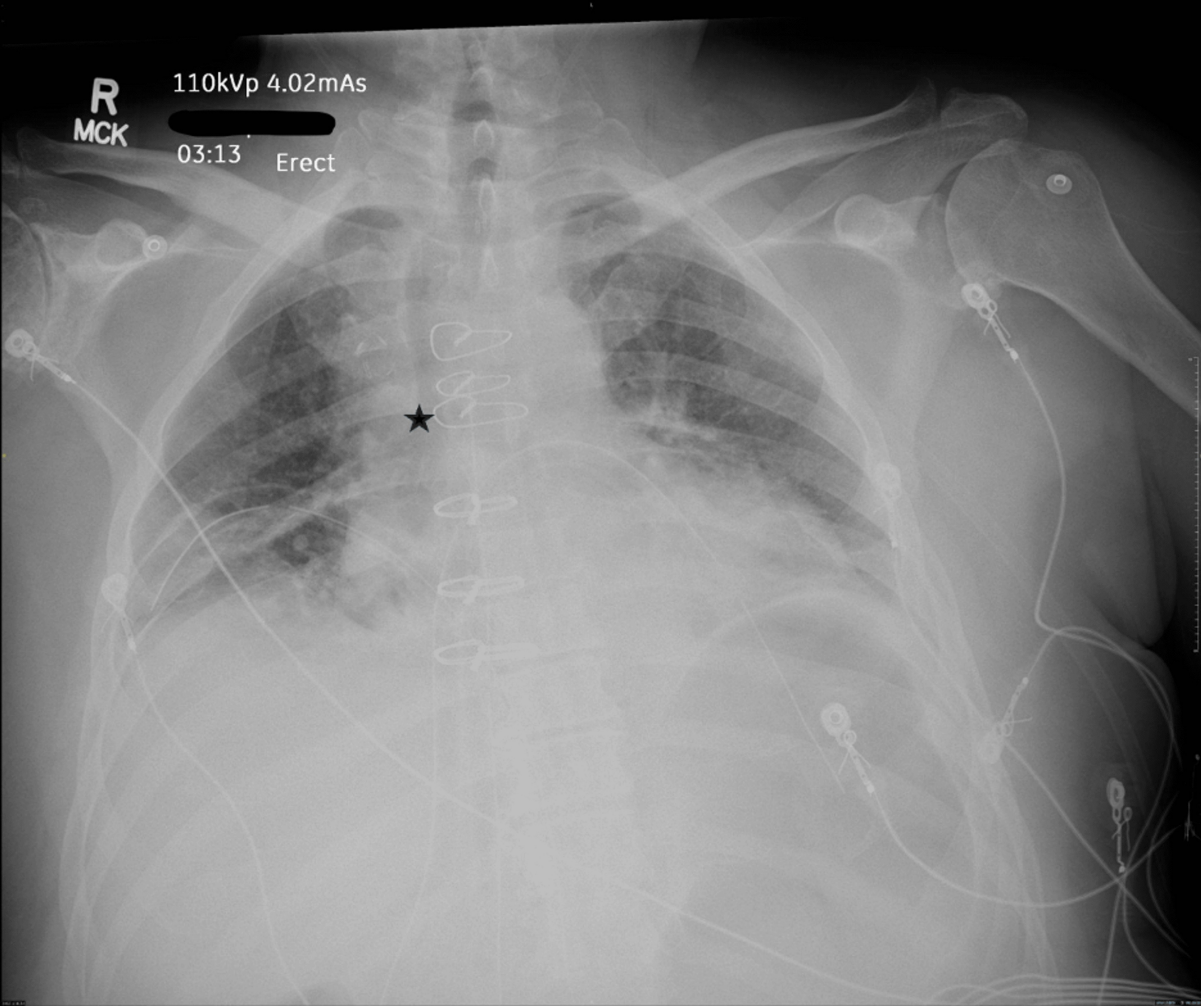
Post-operative chest X-ray on post-operative day one demonstrating six sternotomy wires after thymectomy, one of which is denoted by the star
